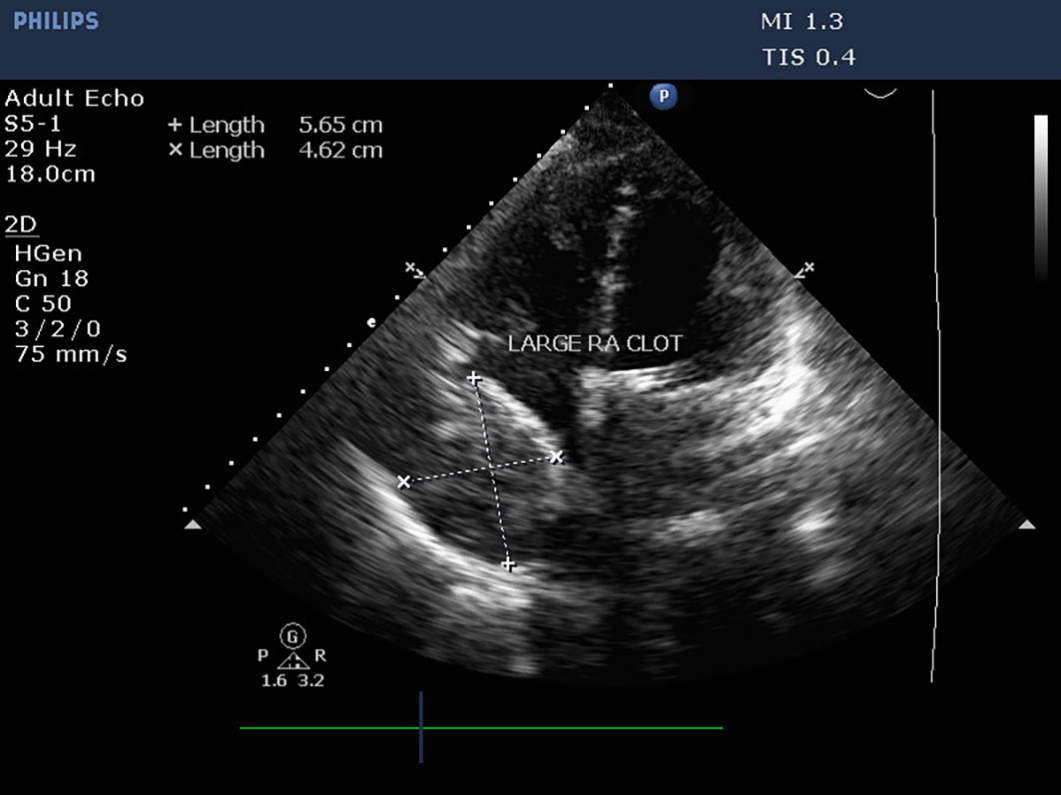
Transthoracic echocardiography demonstrating a large mass occupying the RA cavity after MVR.
